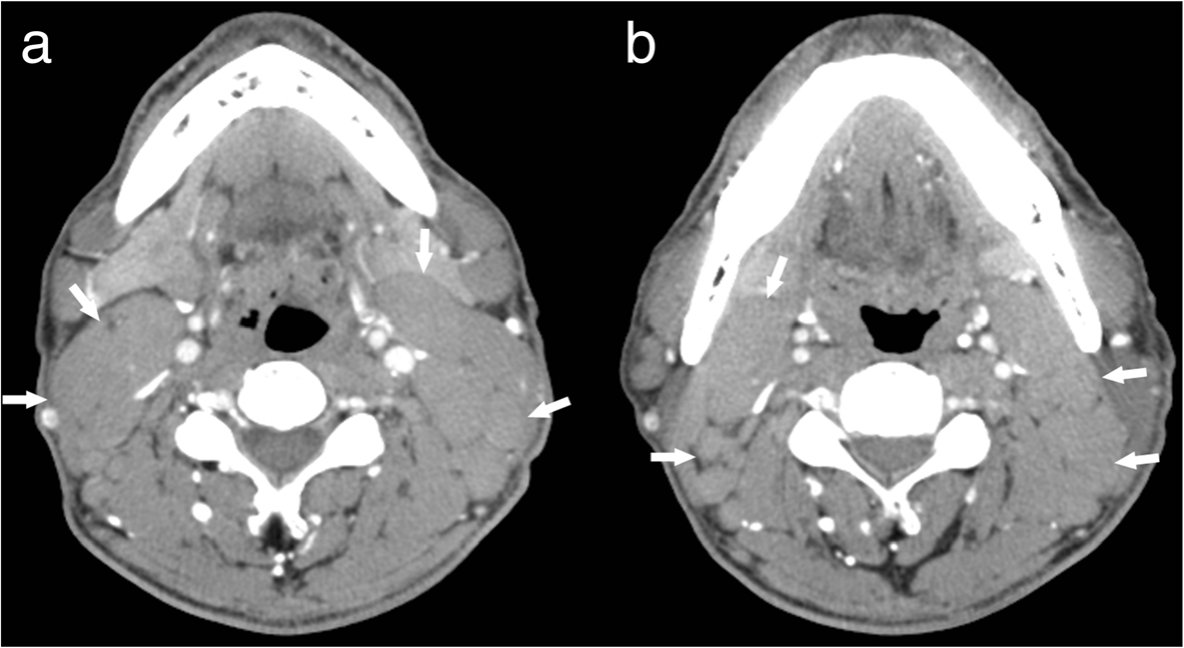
A 35-year-old man who presented with non-Hodgkin’s lymphoma. a-b Contrast-enhanced CT images of the neck show multiple nodules with mild enhancement patterns (white arrows), with the nodules being located on both sides of the neck (white arrows)
